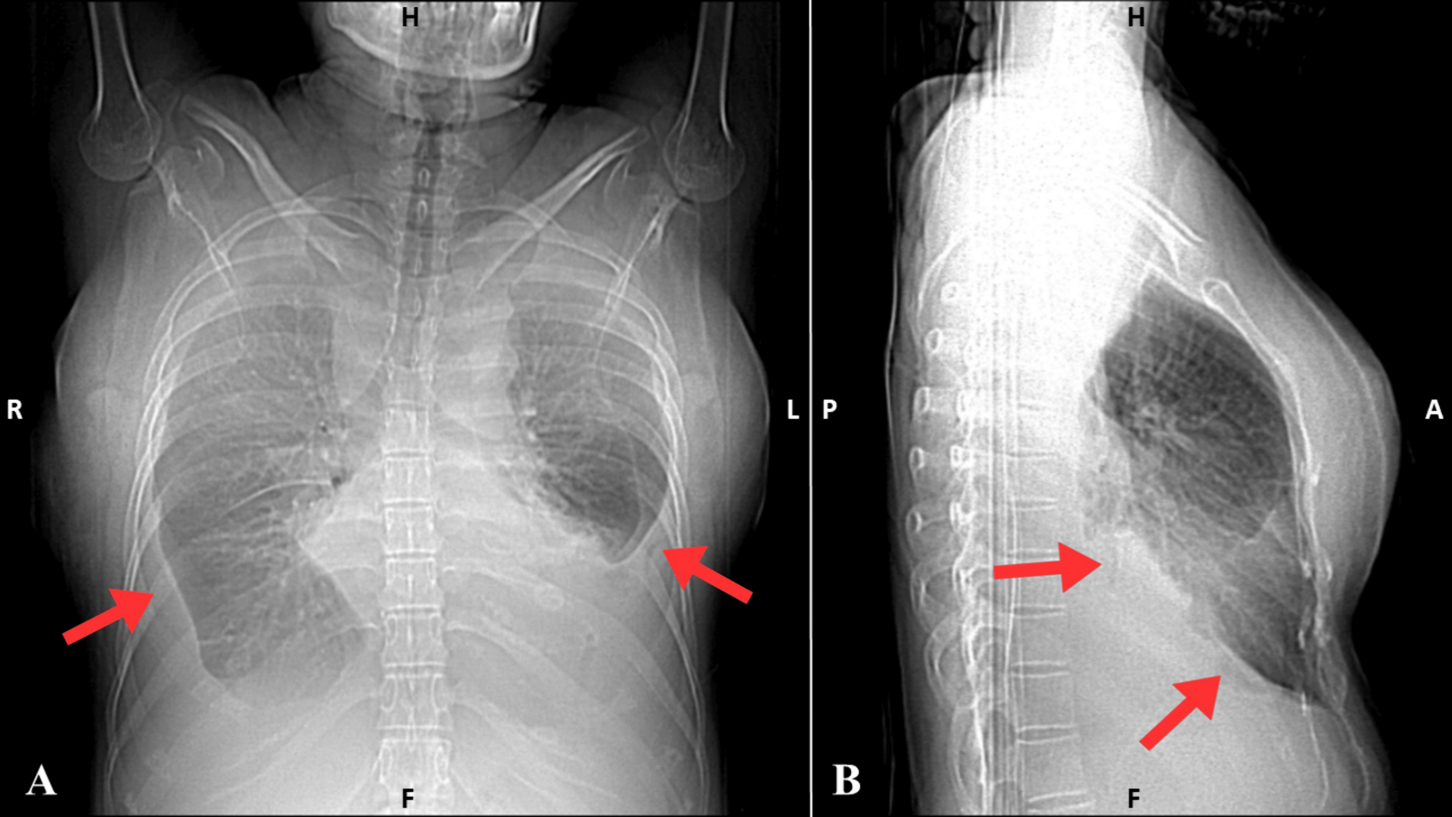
Chest X-ray post-thoracentesis (panels A and B) showing residual pleural effusion (March 30, 2025). A: Anteroposterior (AP) view showing bilateral residual pleural effusions (red arrows) with partial re-expansion of the lungs. B: Lateral view showing residual pleural effusion (red arrows) post-thoracentesis.
